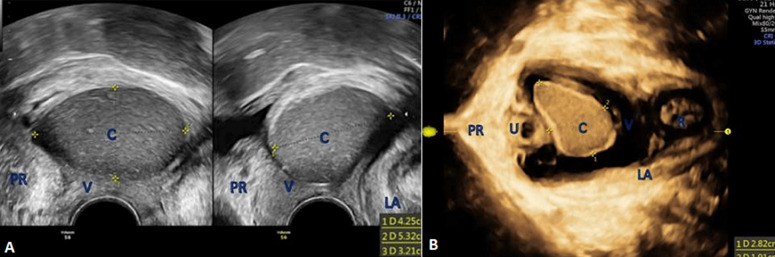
pelvic floor ultrasound (PFUS) evaluation of the cystic mass; a well-defined, round, thick-walled unilocular cystic lesion containing sub-echogenic contents without a septum, originating at the right lateral vaginal wall, extending from the introitus to the cervix of the uterus: A) 2D PFUS from patient #2; B) 3D PFUS - axial plane from patient #3; U=urethra, LA=levator ani, V=vagina, R=rectum, C=cyst, PR=pubic rami
